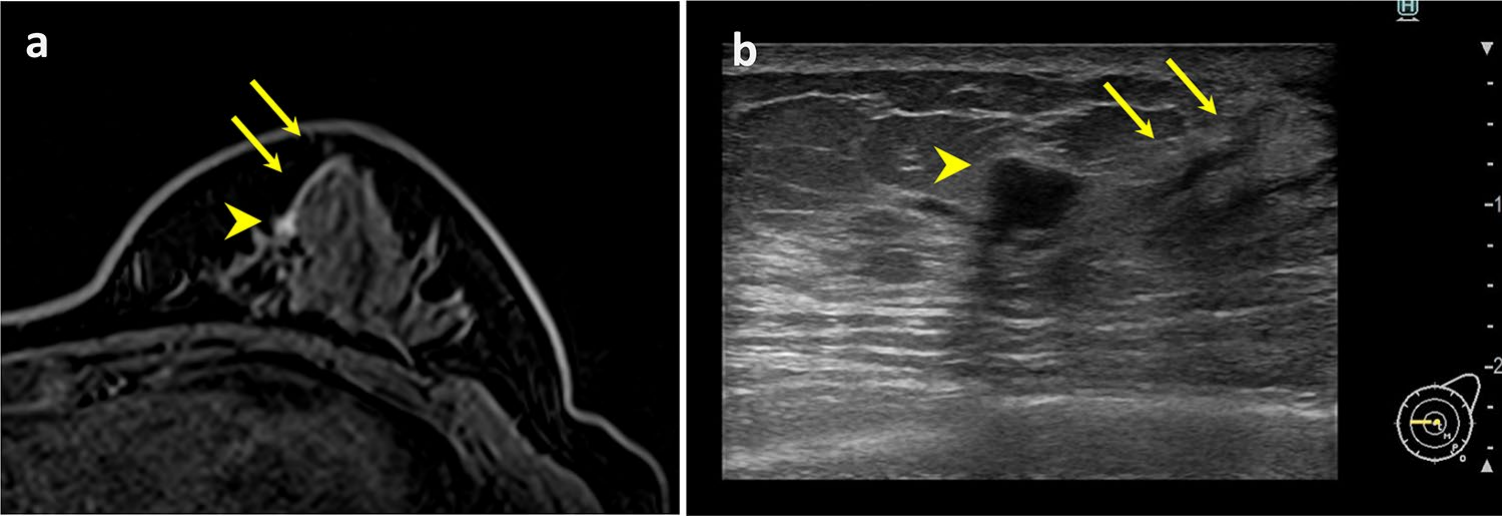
Preoperative MRI and ultrasonography revealed intraductal spread to the nipple. Arrow, intraductal spread to the nipple; arrowhead: cancer lesion. a MRI. b Ultrasonography
